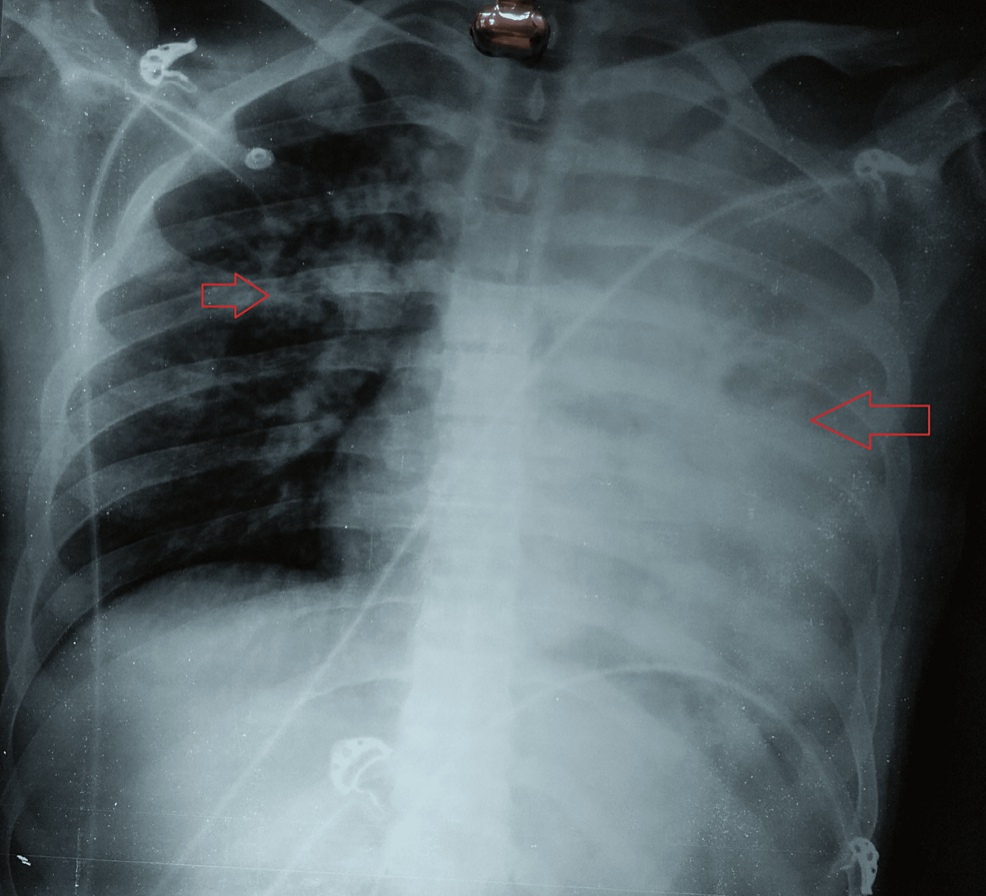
Chest radiograph (P-A view) showing consolidation on the left upper and lower lobes and right upper lobe
